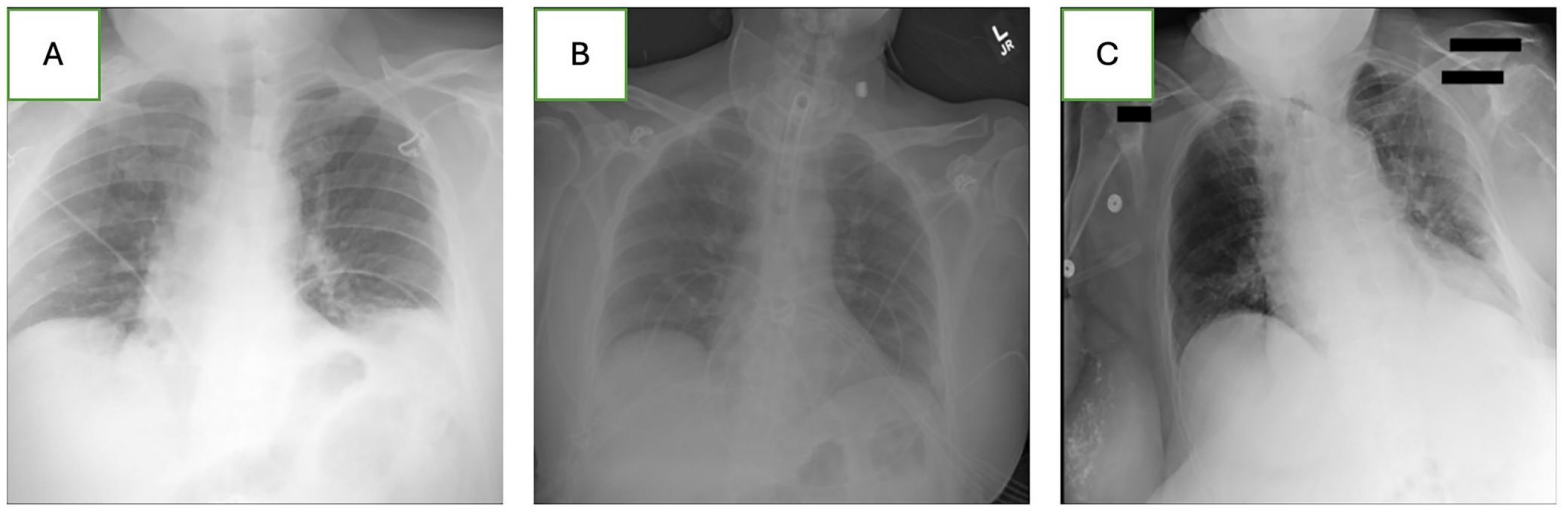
Three samples of the radiographs in which the model had poor performance. (A) Parts of the chest radiograph that are important to the model are missing from the image. eSFV vs SFV: 1525 cm^3^ vs 6320 cm^3^, eVFV vs VFV: 660 cm^3^ vs 4202 cm^3^, eSMI vs SMI: 150 cm^3^/m^2^ vs 1003 cm^3^/m^2^ B. This chest radiograph is dark and does not encompass some important regions for prediction. eSFV vs SFV: 6234 cm^3^ vs 1741 cm^3^, eVFV vs VFV: 3153 cm^3^ vs 5234 cm^3^, eSMI vs SMI: 929 cm^3^/m^2^ vs 1286 cm^3^/m^2^ C. The patient is rotated, and the lungs are not properly inflated. eSFV vs SFV: 4003 cm^3^ vs 5940 cm^3^, eVFV vs VFV: 2046 cm^3^ vs 3151 cm^3^, eSMI vs SMI: 2501 cm^3^/m^2^ vs 1050 cm^3^/m^2^. (e)SMI = (estimated) skeletal muscle index, (e)SFV = (estimated) subcutaneous fat volume, (e)VFV = (estimated) visceral fat volume.
